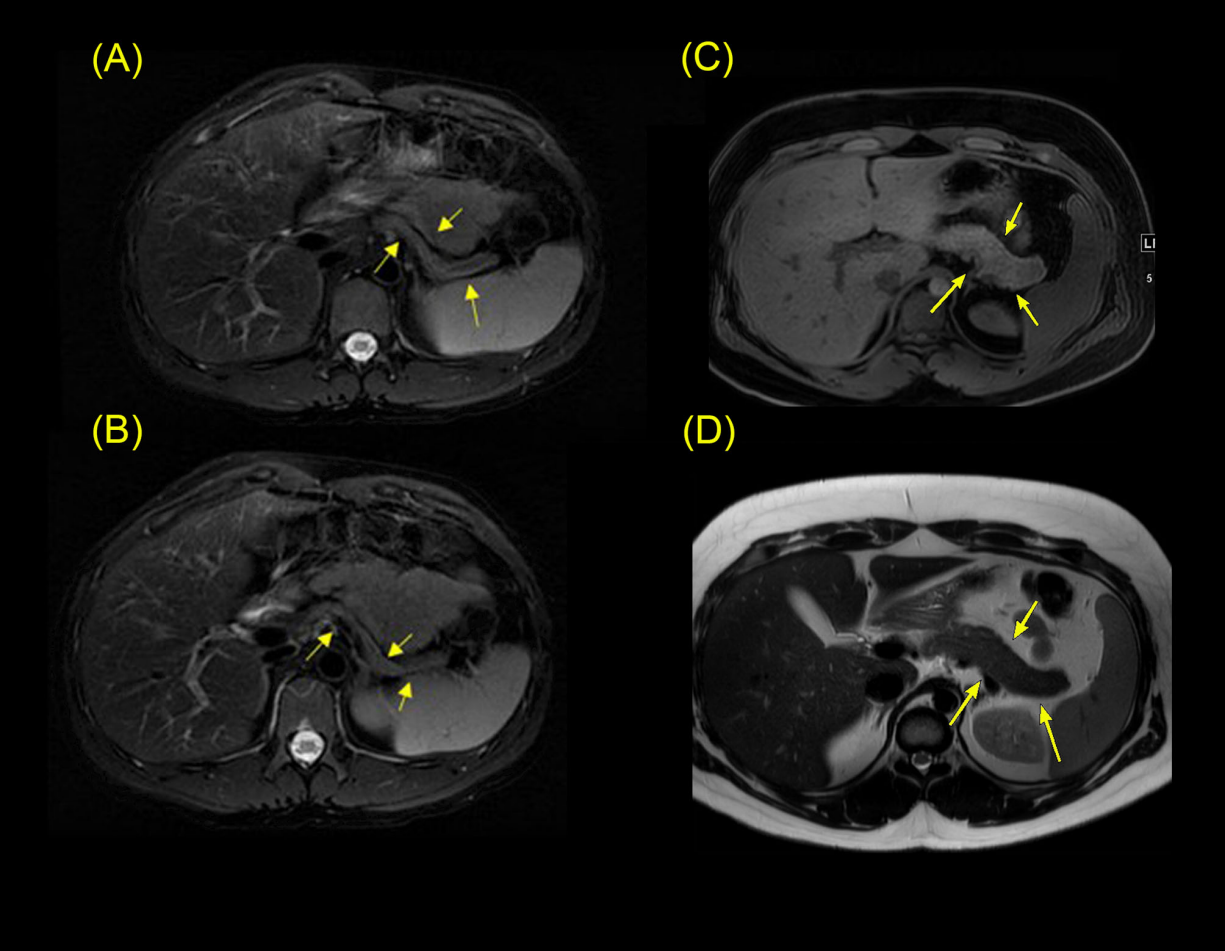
Pancreatic MRI scan of the index patient (patient A) and control patient. The MRI scan in (A, B) showing a small atrophic pancreas of the index patient (patient A). Images clearly show global parenchymal atrophy of the pancreas. The pancreatic parenchyma appears thin with heterogeneous low T1 signals, indicating fibrosis. The MRI scan in (C, D) are axial T2 weighted MRI images of a control case, a 26-year-old male with type 1 DM. The images (C, D) show a normal volume of the pancreas.
